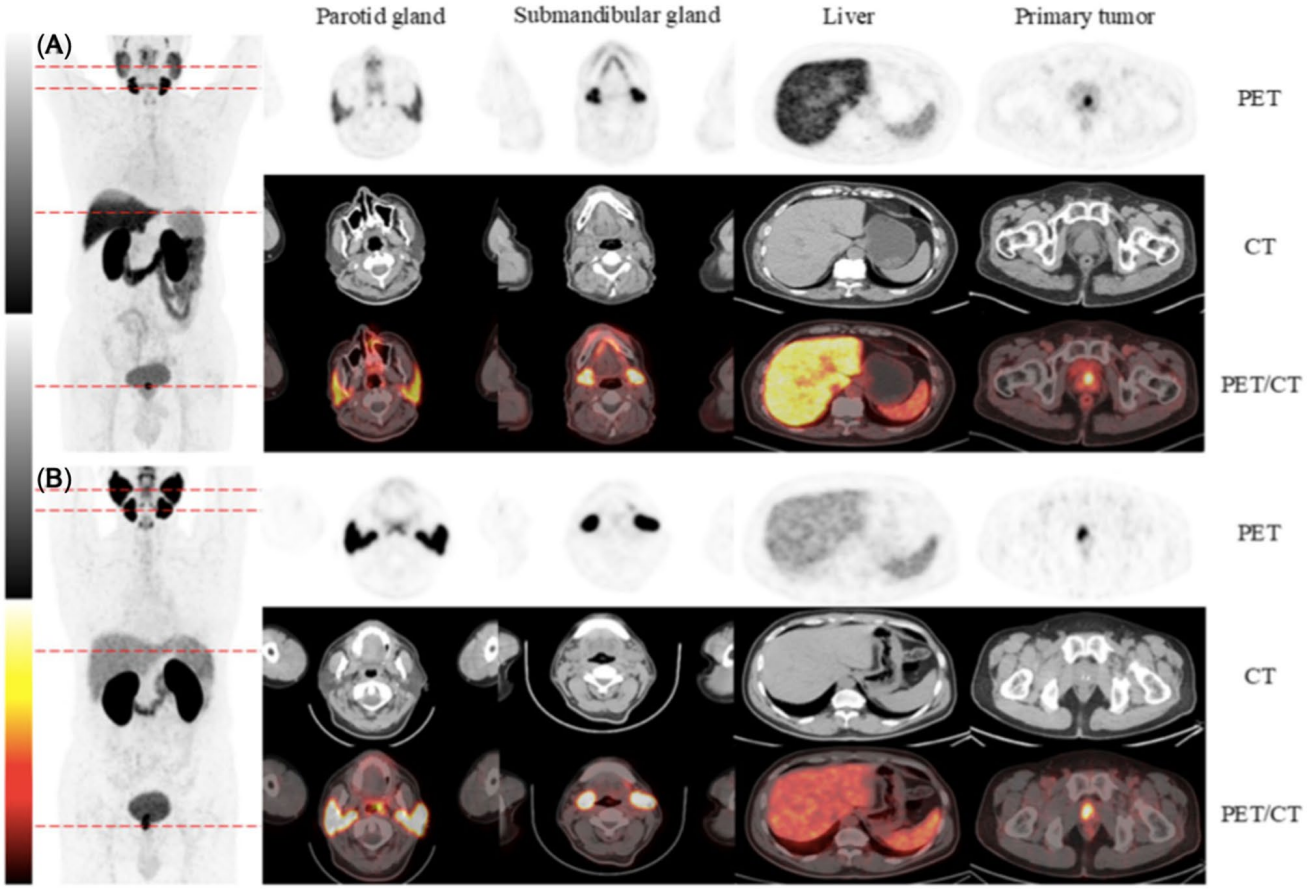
Representative PET/CT images acquired 2 h post-injection of ^64^Cu-labeled PSMA ligands in two patients with primary PCa. Panel (A) shows MIP, low-dose axial CT, and fused PET/CT images of an 80-year-old patient (PSA 10.3 ng/mL, Gleason score 4 + 3) after administration of [^64^Cu]Cu-DOTA-PSMA-3Q, with notable uptake in the parotid gland (SUV_max 7.49), submandibular gland (12.97), liver (8.09), spleen (4.36), and primary tumor (14.87). Panel (B) shows corresponding images from a 65-year-old patient (PSA 18.49 ng/mL, Gleason score 4 + 3) imaged with [^64^Cu]Cu-NOTA-PSMA-3Q, demonstrating uptake in the parotid gland (19.83), submandibular gland (24.97), liver (4.57), spleen (4.76), and primary lesion (14.46). Reproduced from Liu et al. [110], licensed under CC BY-NC-ND 4.0 (https://creativecommons.org/licenses/by-nc-nd/4.0/)
